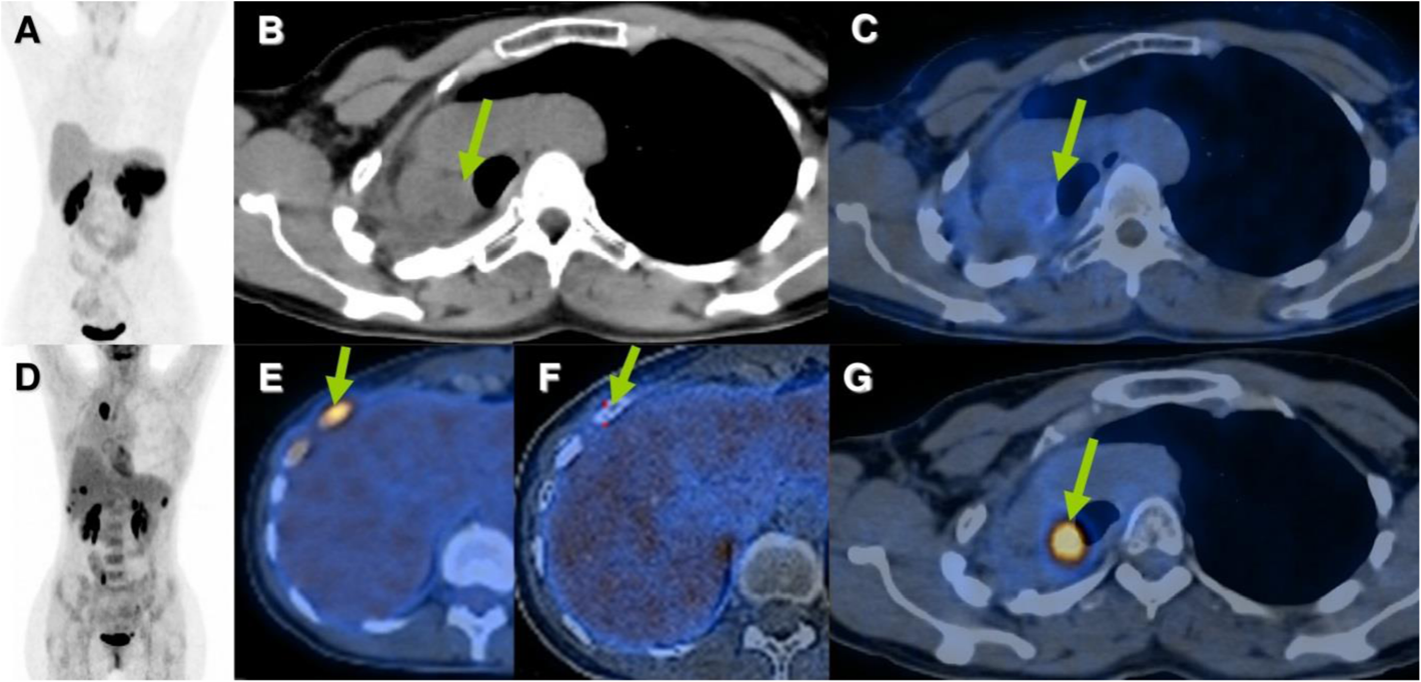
Atypical carcinoid of the lung, first diagnosed in 03/2000. Following upper and middle lobe resection of the right lung (03/2000) and external beam irradiation therapy with 70 Gy (06/2007), the patient underwent multiple operations for residual tumor. Patient was referred for restaging with Ga-68 DOTATOC and FDG PET/CTs (result not discussed in the text). Both the PET/CTs performed in 2010 showed somatostatin receptor-negative, FDG-positive local residual tumor and bone metastases. a Ga-68 DOTATOC PET MIP image. b CT axial slides. c, f Ga-68 DOTATOC PET/CT fused axial images. g, e FDG PET/CT fused axial images. d FDG PET MIP image
